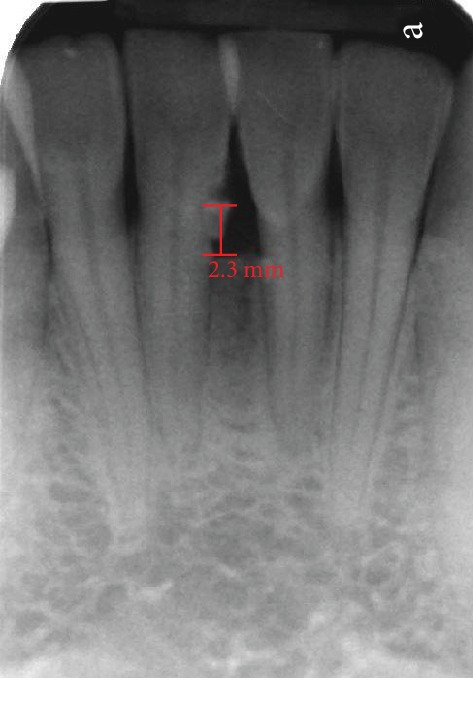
Radiographically 2.3 mm bone loss was noted between #24 and 25.
